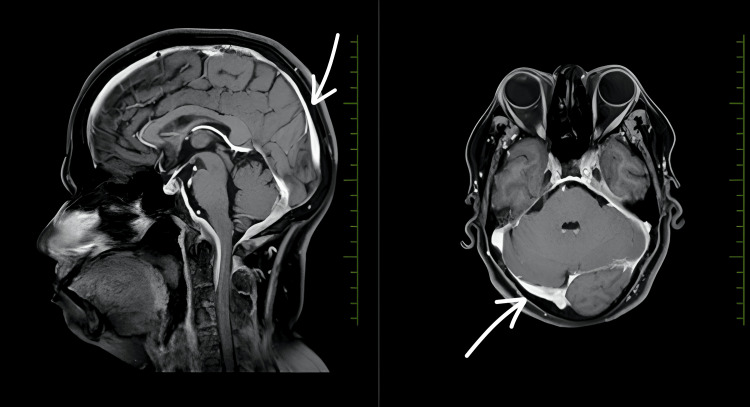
MRI with contrast sagittal and axial view. There is smooth dural thickening and enhancement of pachymeninges along the mid and post-cranial fossa. Anteriorly: Extending from the clivus superiorly to the tectorial membrane and dura of the spinal cord at the level of the C2 vertebra. Posteriorly: Extending along the bilateral tentorium cerebelli (left > right) and falx cerebelli.
